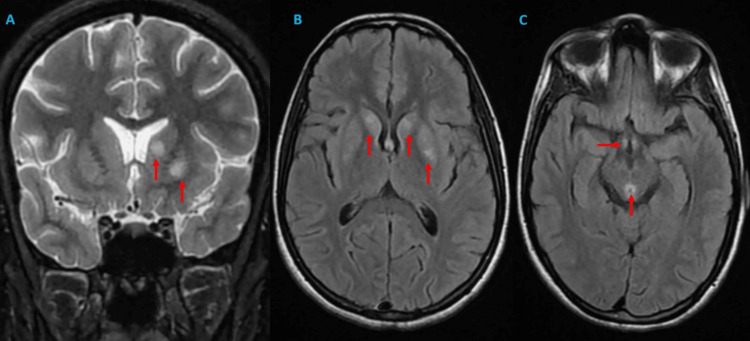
A and B: Coronal T2-weighted and axial FLAIR MRI images revealed bilateral hyperintensities (red arrows) in the caudate nuclei, along with an atypical hyperintense lesion in the left lenticular nucleus (putamen). Such basal ganglia involvement is uncommon but has been documented in non-alcoholic cases of Wernicke encephalopathy. C: Axial FLAIR MRI image demonstrates symmetric hyperintensities in the mammillary bodies and periaqueductal gray matter (red arrows), consistent with classic manifestations of thiamine deficiency. MRI: magnetic resonance imaging, FLAIR: fluid-attenuated inversion recovery
